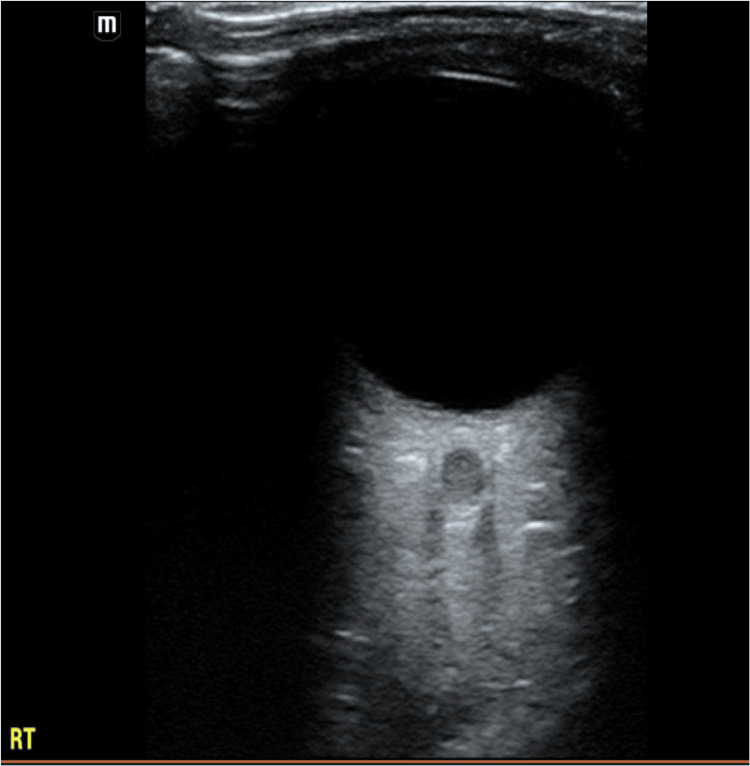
Bedside ocular ultrasound of the right eye showing a normal optic nerve for comparison.
